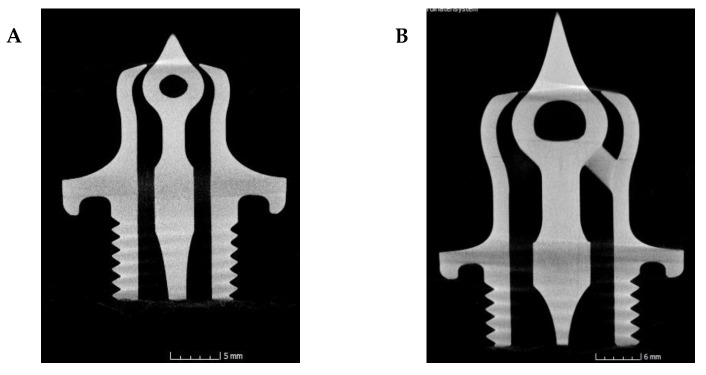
CT images of 2.5 N (A) and 10 N (B) alumina nozzles.
